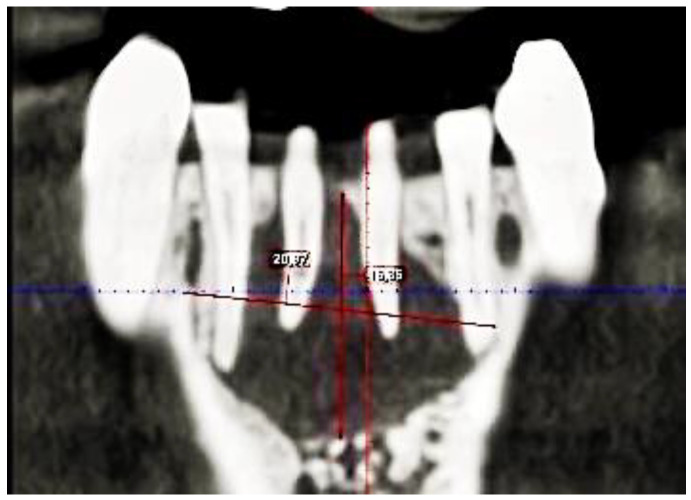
A frontal image of the CBCT taken on the first appointment showing bone resorption (with a diameter of 20.97 mm width and 16.35 mm height) of the cyst in the middle of the lower jaw.
